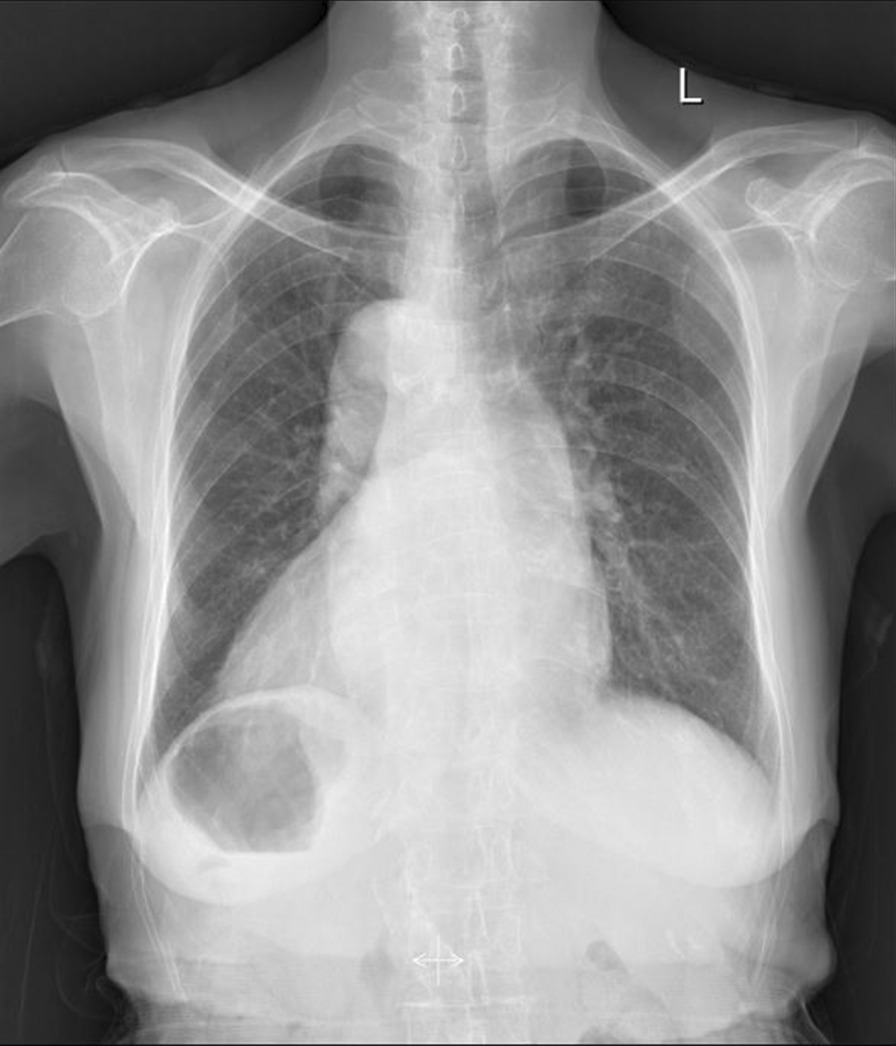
Chest X-ray showed mirror-image dextrocardia, with the heart’s silhouette on the right side of her chest
